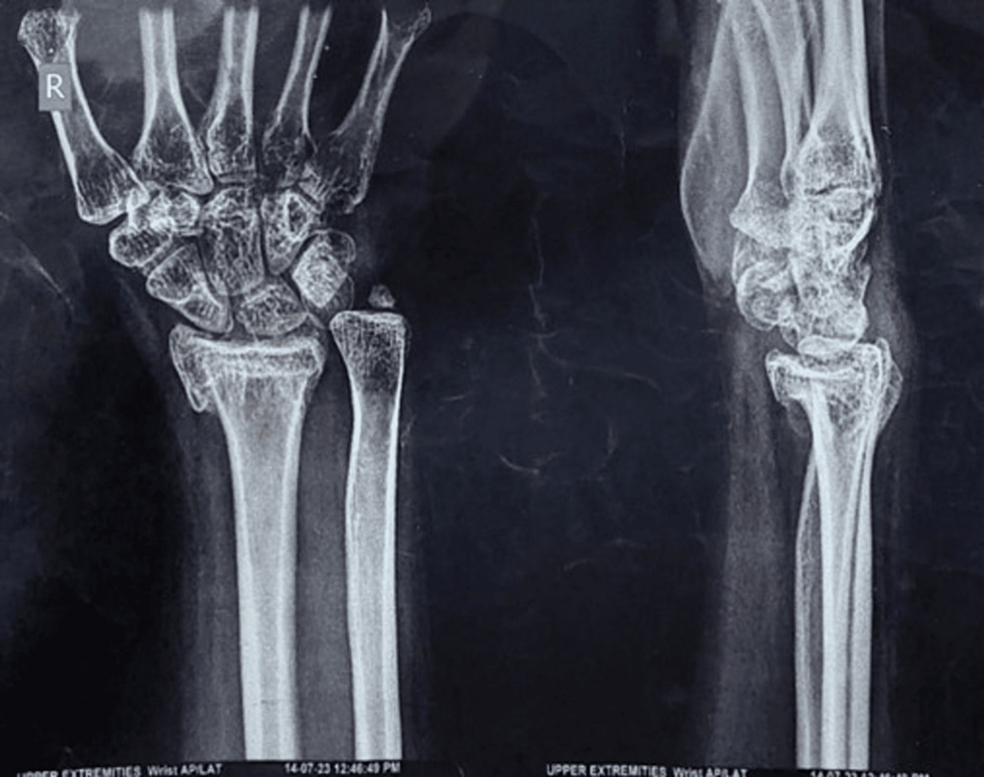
Pre-operative radiograph
